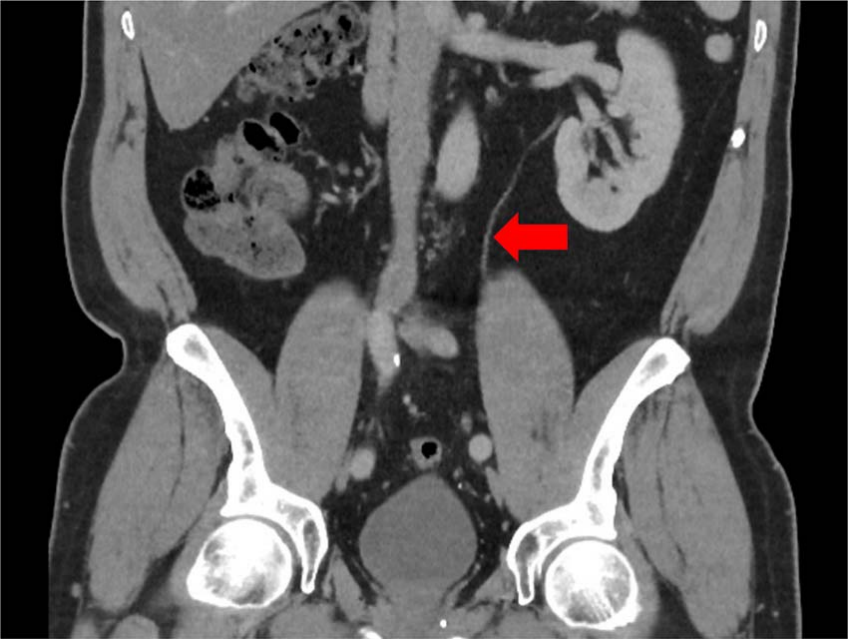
CT scan taken 1 year pre-operatively during a prior episode of acute sigmoid diverticulitis, showing a single ureter (arrow) descending from the left kidney, which entered the bladder at a single ureteric orifice.
